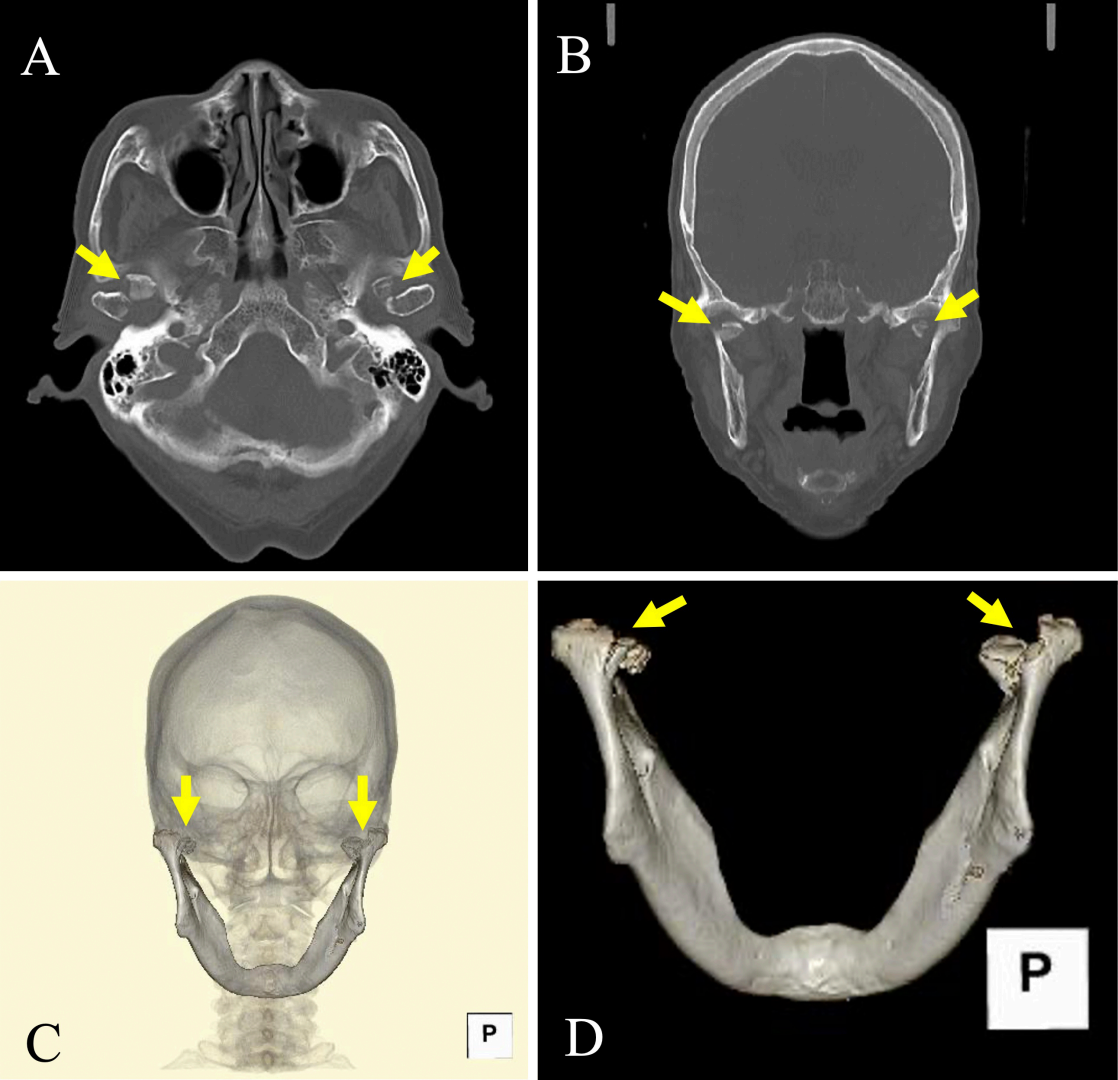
Computed tomography images. (A) Axial image. (B) Coronal image. (C) Three-dimensional (3D)-reconstructed image. (D) Mandibular 3D-reconstructed image. Arrows indicate fracture lines.
